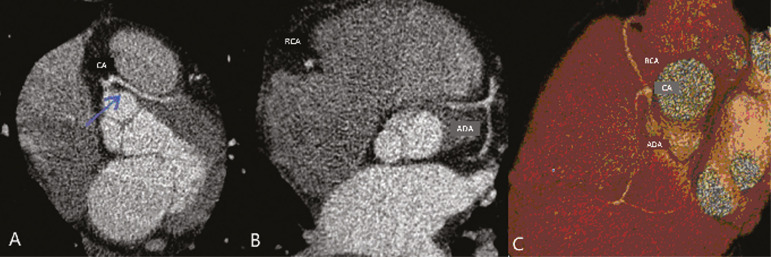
Right pulmonary nodule (blue arrow) on CTA.
